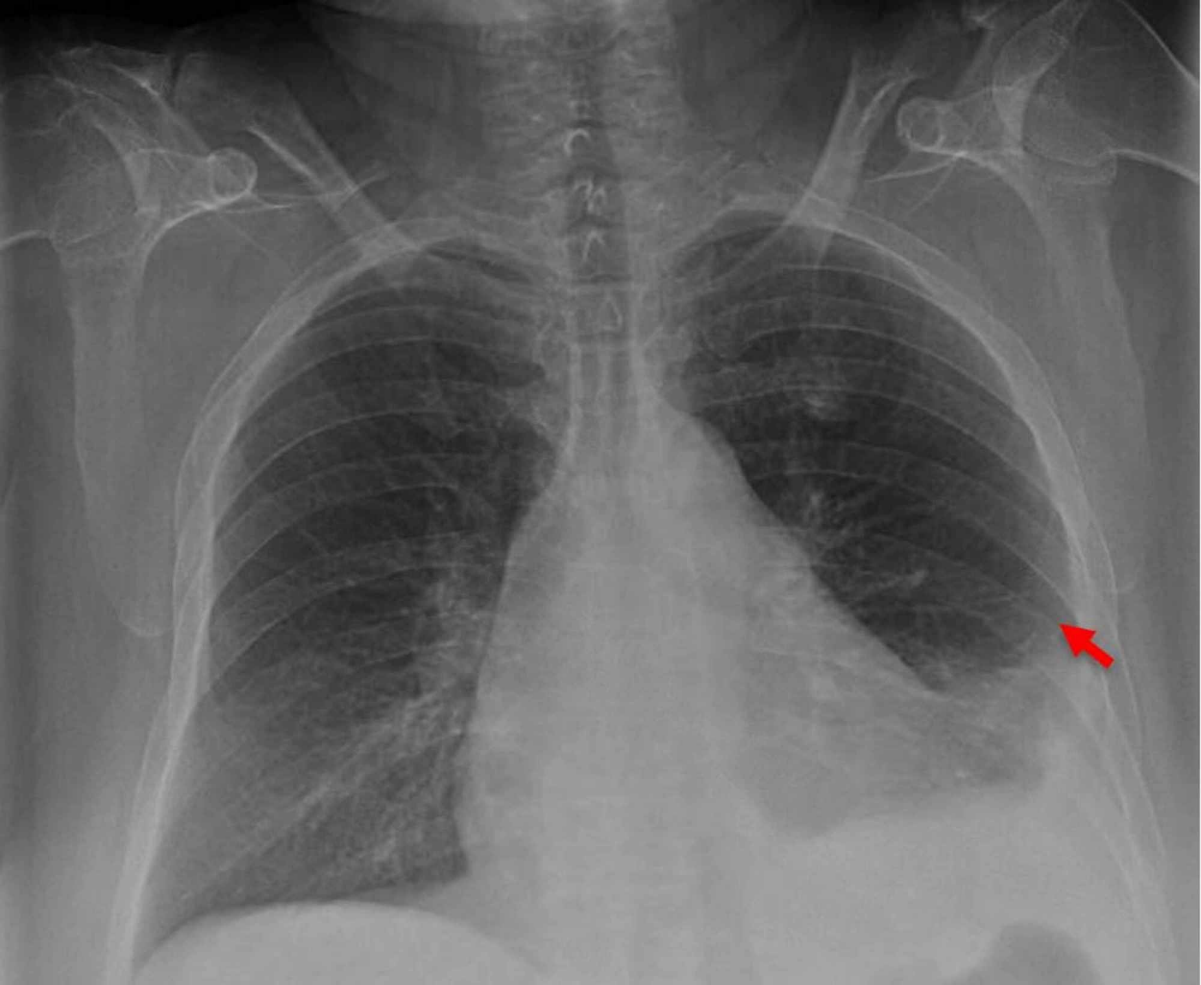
Chest x-ray showing development of left pleural effusion (red arrow).
